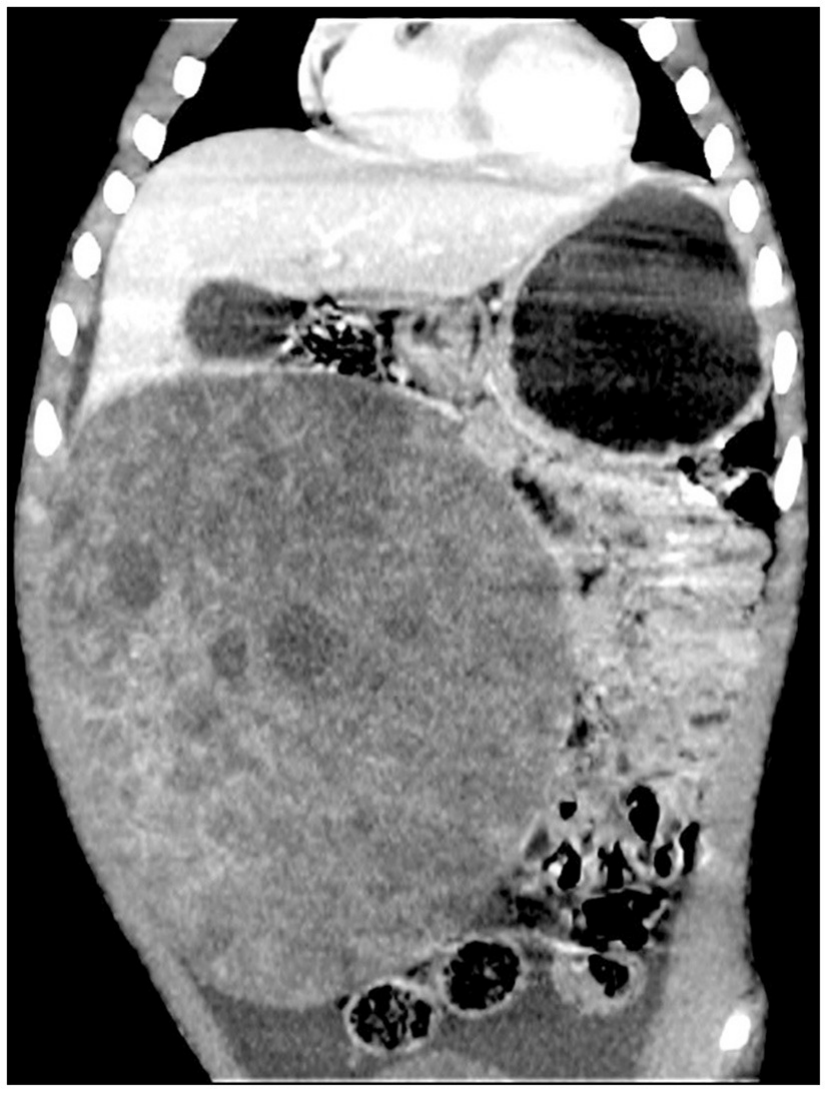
Enhanced CT of three-dimensional reconstruction. The adjacent right branch of the proper hepatic artery, abdominal aorta, right common iliac artery, and mesenteric vein was displaced by compression, and the distal branches of the hepatic artery were penetrated within the tumor.
